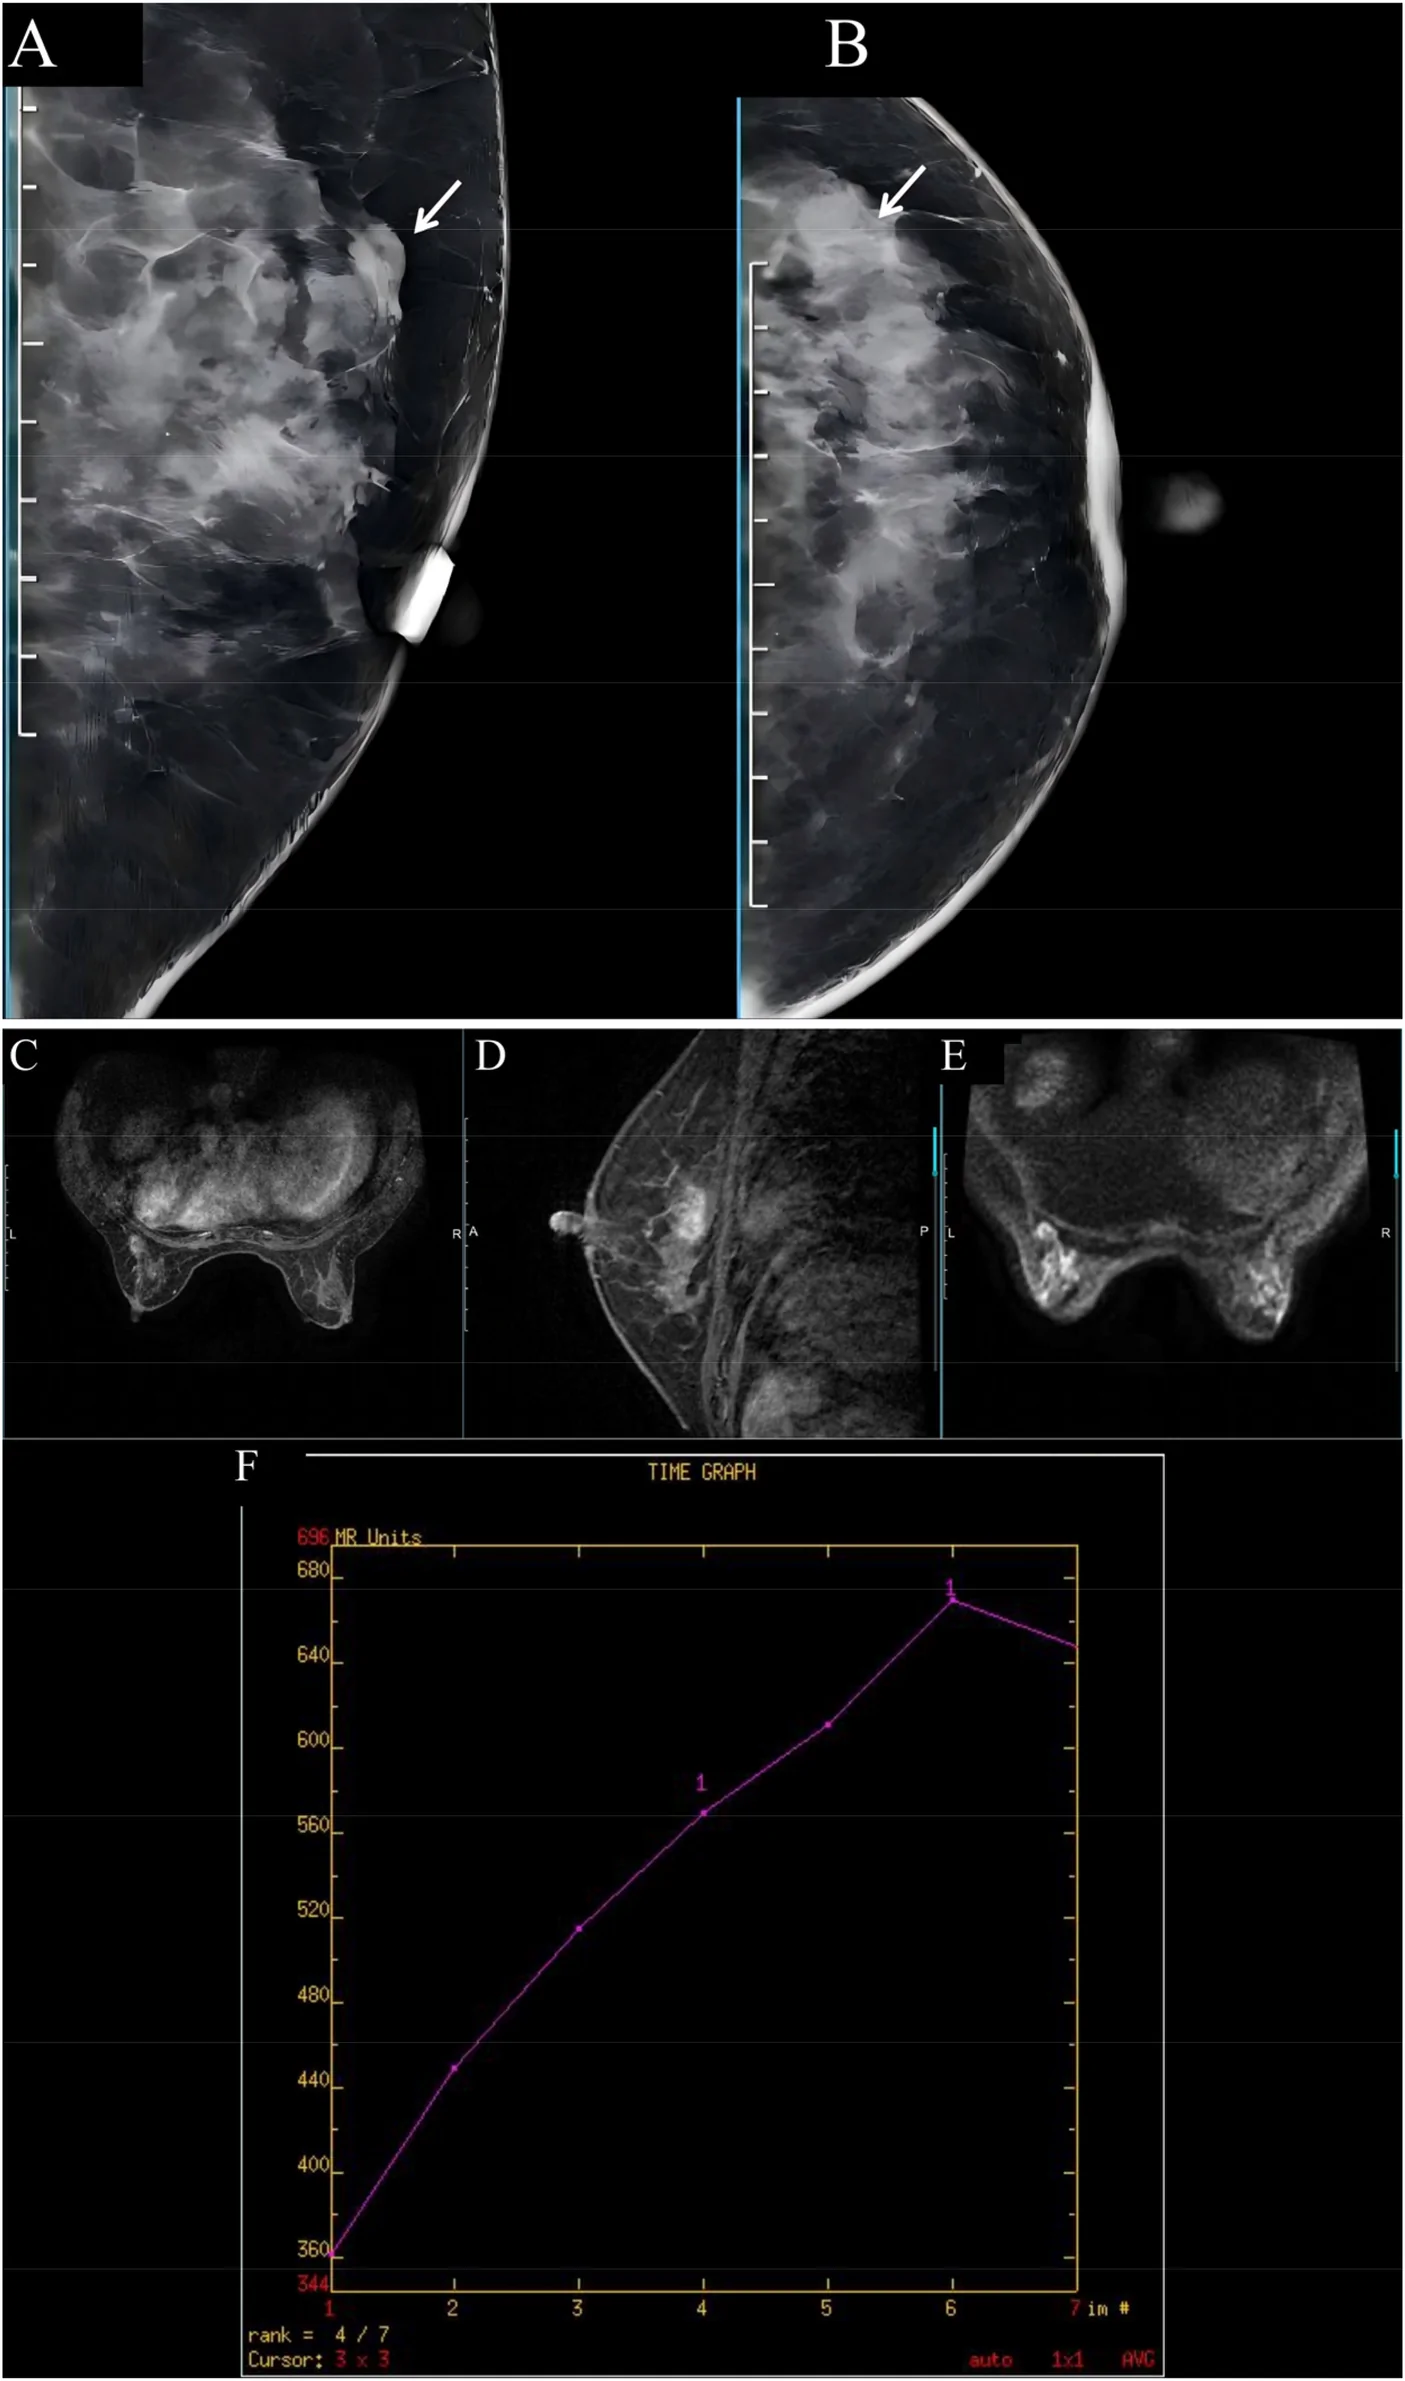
Mammographic and magnetic resonance imaging findings of the left breast in 2024. (A,B) Craniocaudal and mediolateral oblique mammograms show an irregular mass with a lobulated contour and indistinct margins in the outer breast (arrows), without definite calcification; the breasts are heterogeneously dense (composition category C). (C,D) Axial and sagittal dynamic contrast-enhanced MRI show an irregular non-circumscribed mass involving the outer breast with heterogeneous internal enhancement. (E) Diffusion-weighted imaging shows heterogeneous high signal; diffusion restriction could not be confirmed because the ADC map and numerical ADC value were unavailable. (F) The time-signal intensity curve shows predominantly progressive enhancement with a slight late decline.
